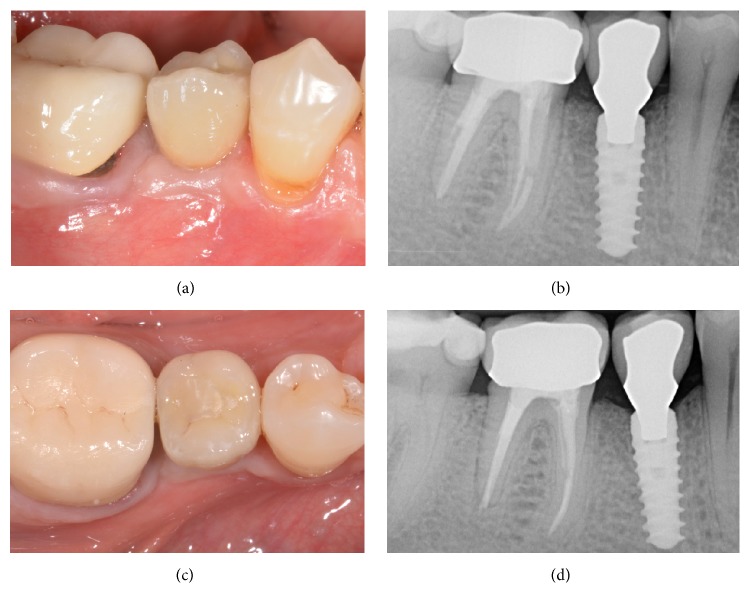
Immediate implant placement of a mandibular second premolar (#45). Final metal-ceramic crown at delivery, lateral view (a); periapical radiograph of the implant at the delivery of the final crown (b); final metal-ceramic crown at delivery, occlusal view (c); periapical radiograph 2 years after implant insertion (d).
